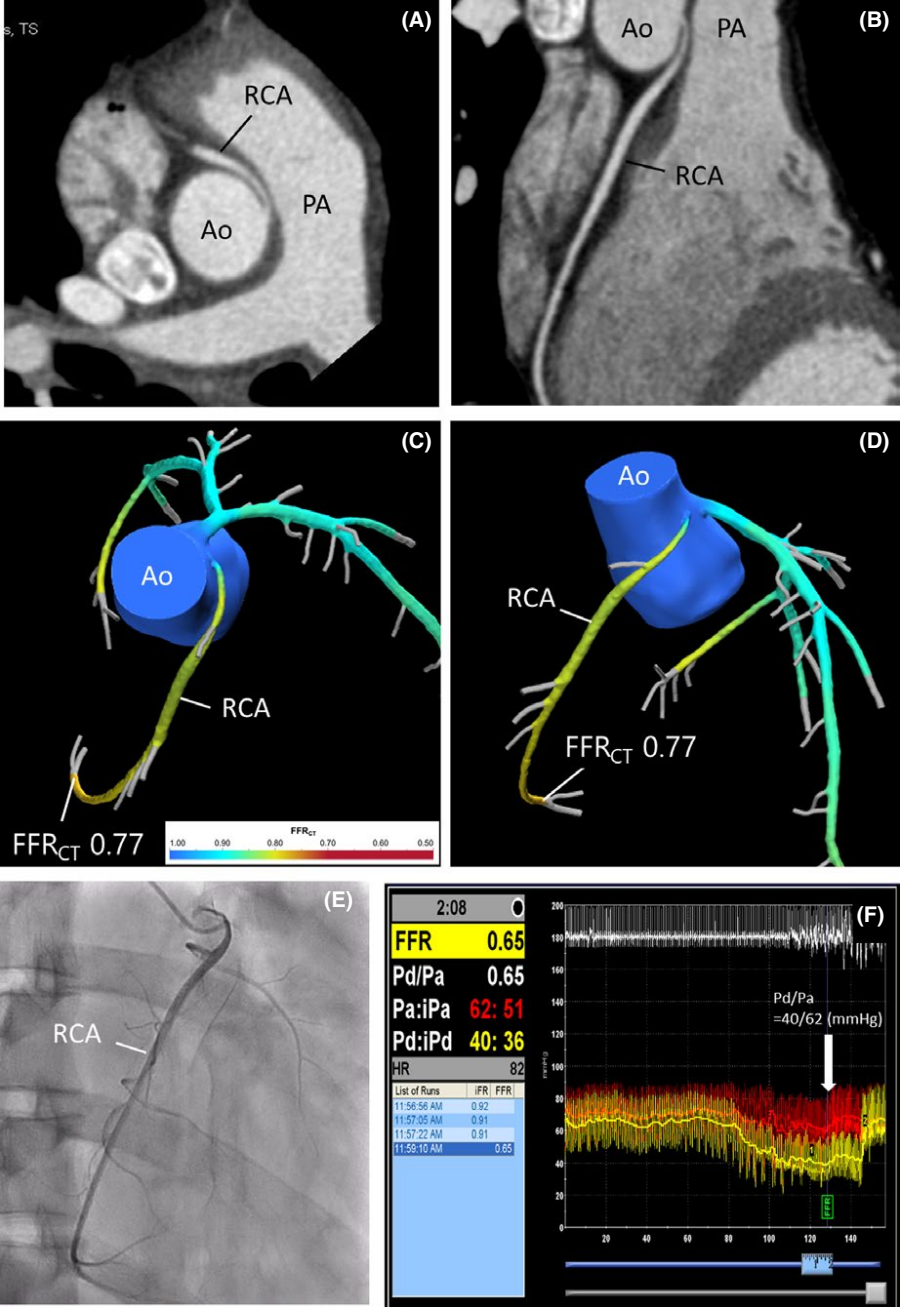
A‐B, Axial and sagittal coronary CT angiography. C‐D, FFR_CT_. E, Coronary angiography, right anterior oblique projection. F, Invasive FFR [= distal coronary/aortic pressures ratio (Pd/Pa)] measured with adenosine 140 μg/kg/min i.v. administration (arrowhead). Ao, aorta; FFR_CT_, CT‐derived fractional flow reserve; PA, pulmonary artery; RCA, right coronary artery
